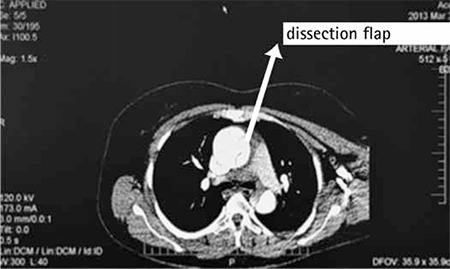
The computed tomography angiography showing the dissection
